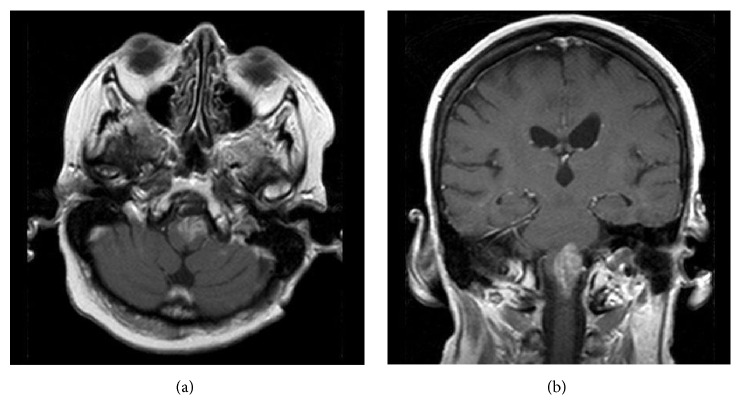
Brain MRI 10 months after surgery. (a) Axial T1-weighted image with gadolinium, showing progression of the enhancing left medullary lesion. (b) Coronal T1-weighted image with gadolinium showing the same medullary lesion.
